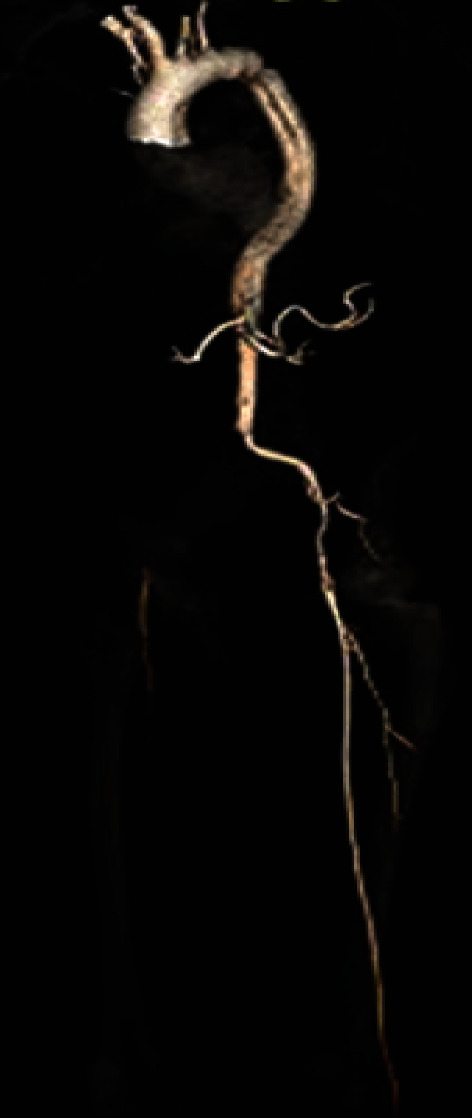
Anterior view of 3D computed tomography (3D CT) imaging of entire aortic artery with obvious dissection of ascending aorta, arch of aorta, right subclavian artery, and right common iliac artery.
